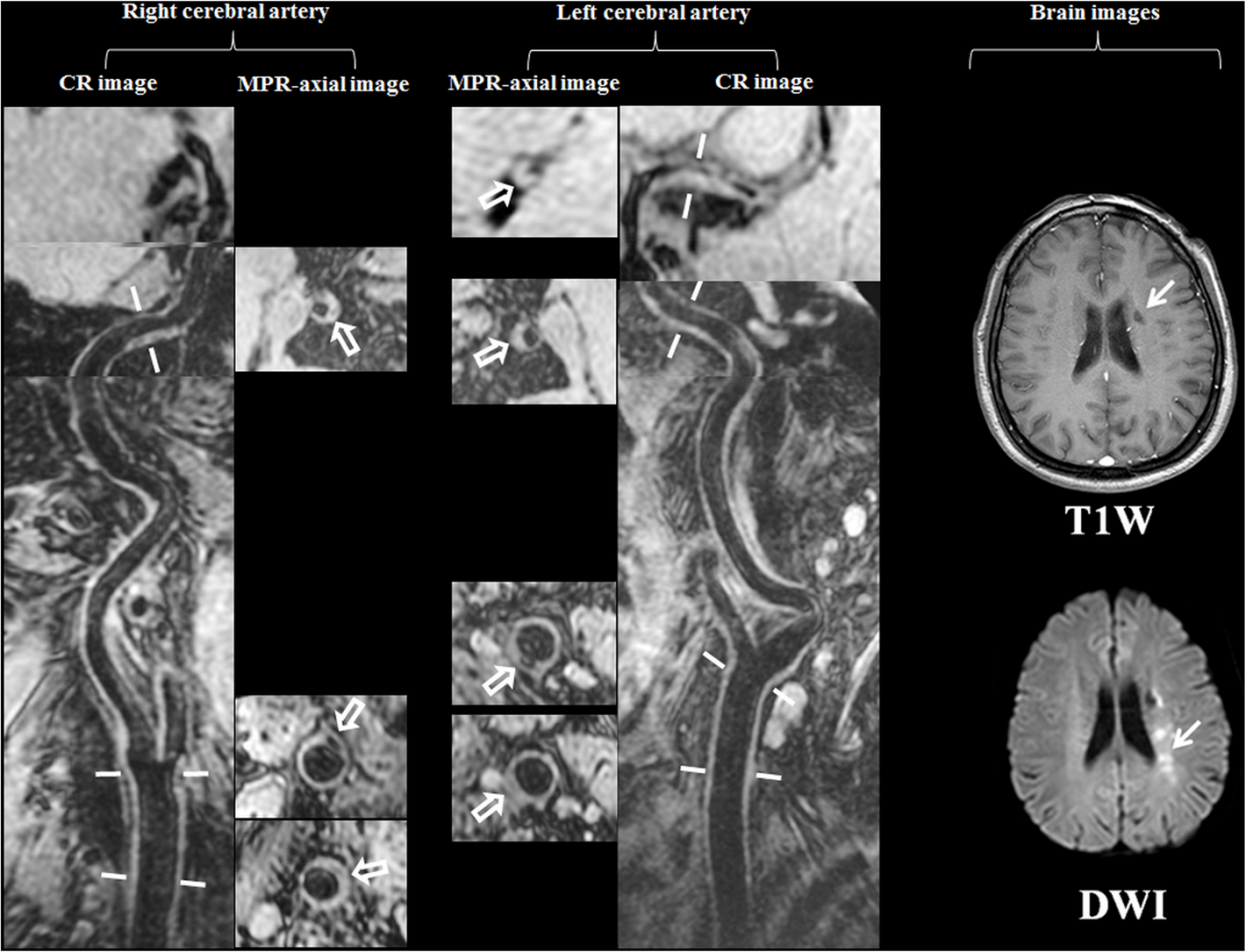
One patient with multiple co-existing intra- and extra-cranial plaques and recurrent stroke. The old infarct showed hypointense on brain T1W image (arrow) and the acute infarct showed hyperintense on brain DWI image (arrow). The multiple plaques can be seen on both left and right curved reconstructed (CR) images of MERGE. Seven plaques (hollow arrows) were identified on axial images after multiple planner reconstruction (MPR) in different cerebral artery segments
